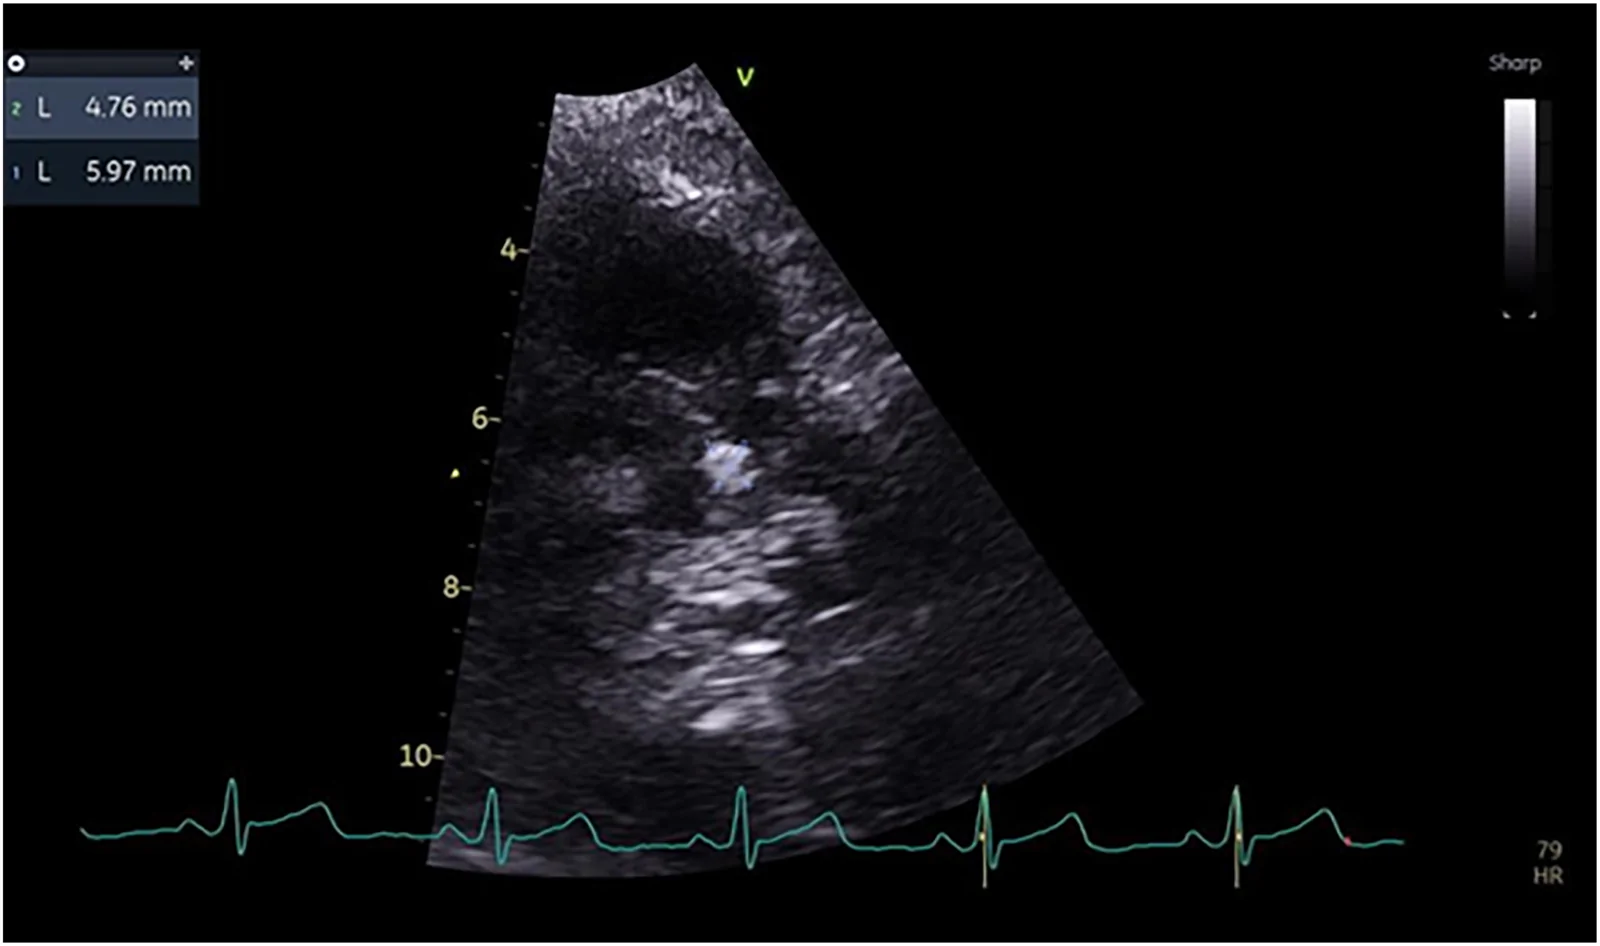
Two-dimensional TTE, basal parasternal short-axis diastolic view at the level of the RVOT obtained at 6 weeks’ follow- up, demonstrates a persistent echogenic mass (4.8 × 6.0 mm) attached to the PV *(red arrow)* consistent with a smaller vegetation.
